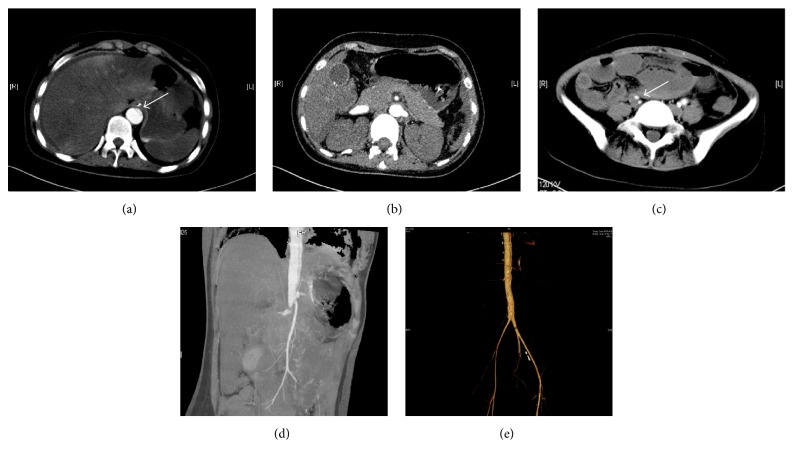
CTA images of aortic dissection. (a) CTA image shows the true and false lumen of aortic dissection and abnormal perfusion of liver and spleen. (b) Decreased perfusion of bilateral kidneys. (c) Abnormal right internal and external iliac arteries. (d and e) 3D reconstruction of CTA images.
